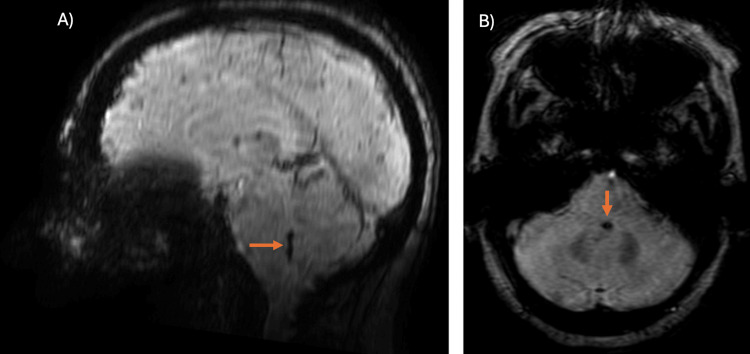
Susceptibility-weighted angiography sequence MRI. (A, B) Sagittal and axial susceptibility-weighted angiography sequence showing hemosiderin remnants in the median sulcus and obex of the fourth ventricle (orange arrows).
